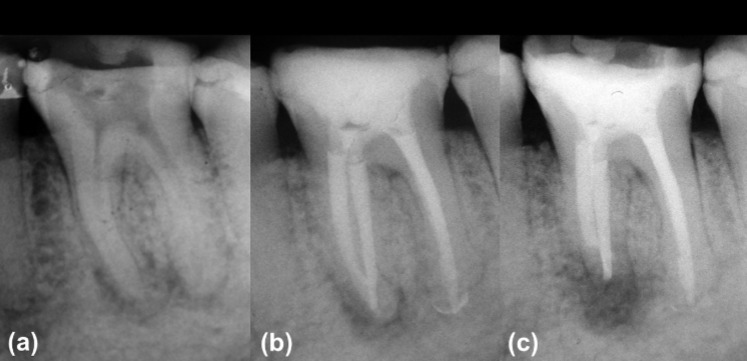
A*) Initial radiograph, *B*) Non-surgical endodontic post-treatment radiography*, C*) Endodontic post-surgery radiography
